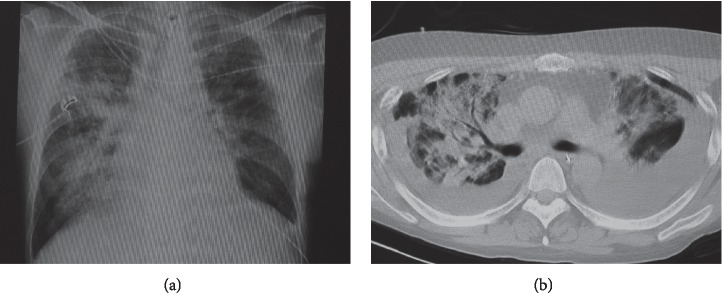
(a) Plain chest radiography showing bilateral infiltrations on the 7^th^ day after surgery. (b) Chest computed tomography imaging showing a significant ground-glass pattern, compatible with acute respiratory distress syndrome.
